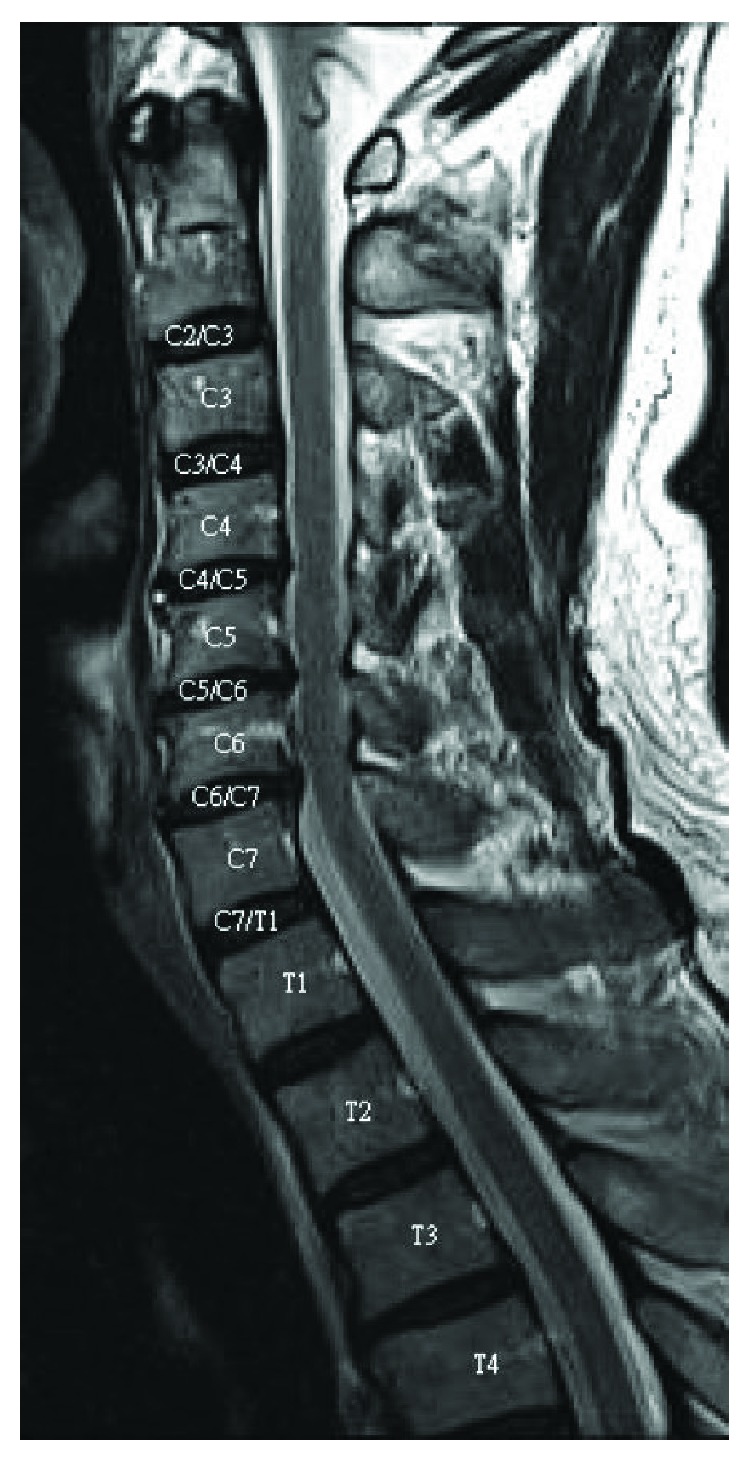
Vertebral body and intervertebral disk detection and labeling in a midsagittal MR image.
